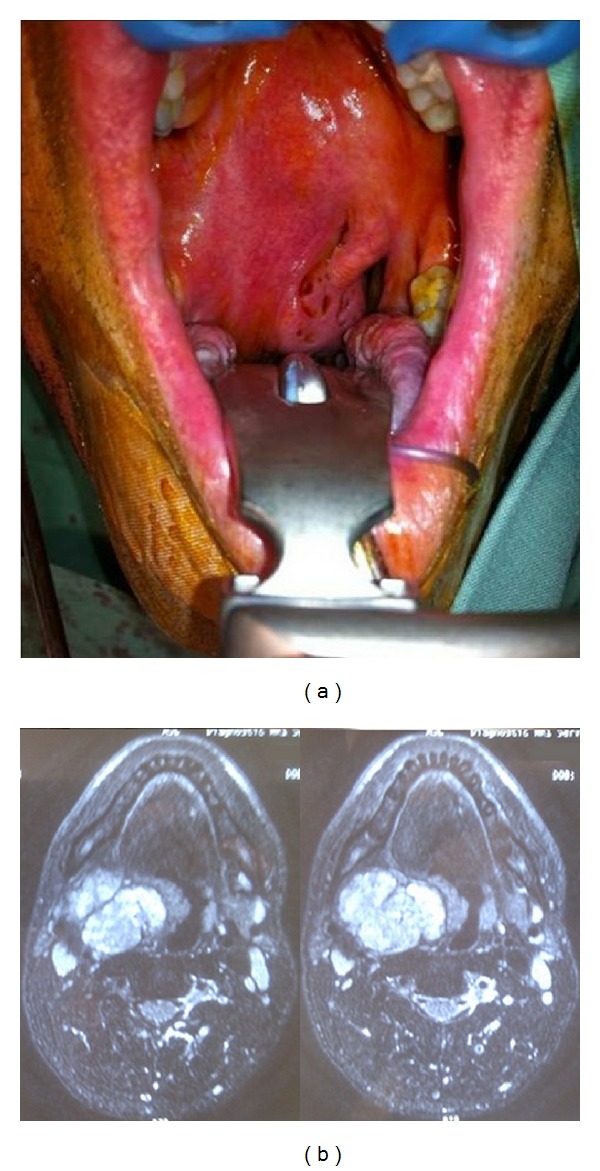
(a) The soft palate is displaced anteromedially by the parapharyngeal mass. (b) Magnetic resonance imaging of the parapharyngeal mass showing a lobulated, homogeneous mass.
